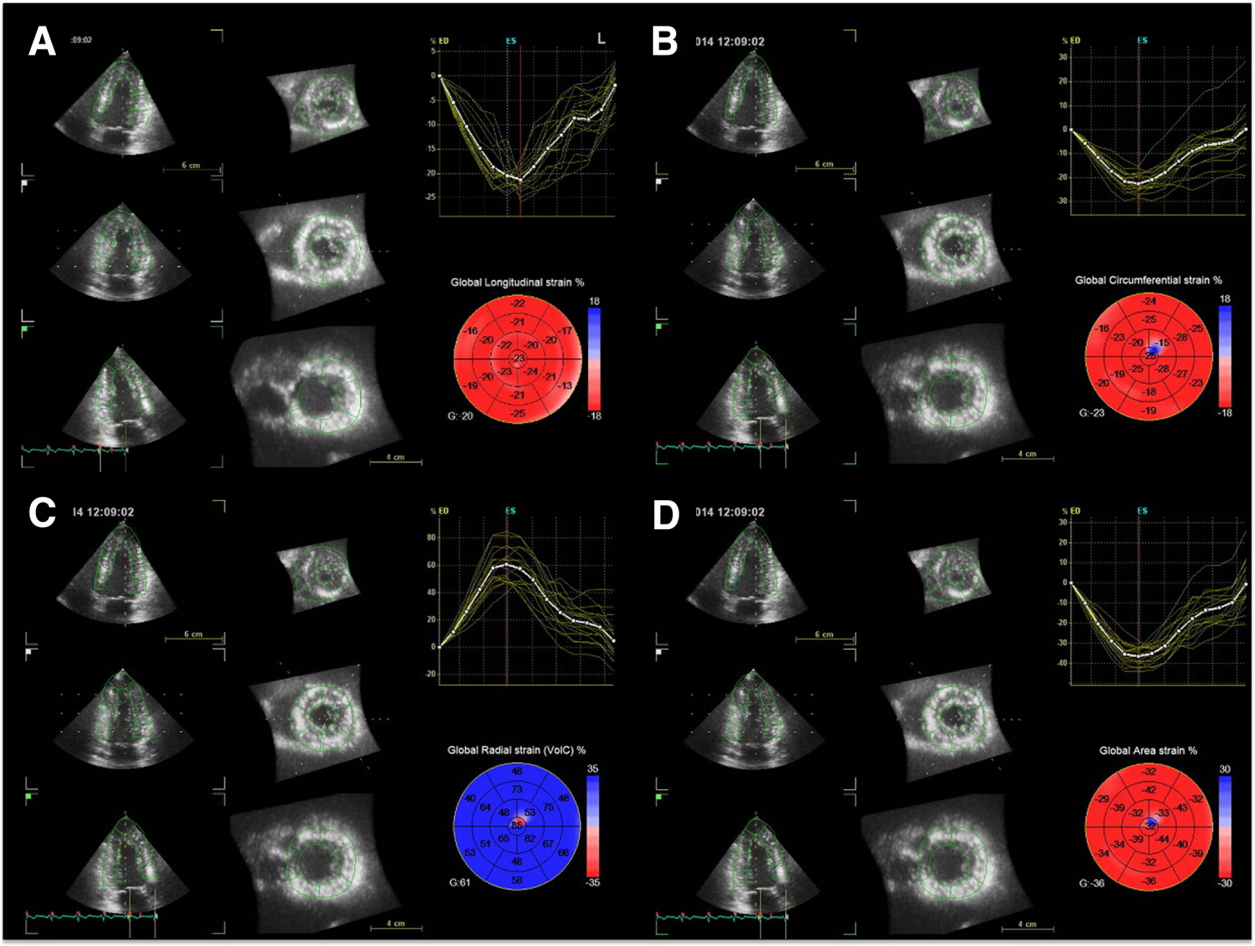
Multiplanar reconstruction images are obtained automatically from single volumetric echocardiographic data. Global longitudinal (A), circumferential (B), radial (C), and area strains (D) can be measured as well as regional segmental values at one analysis.
